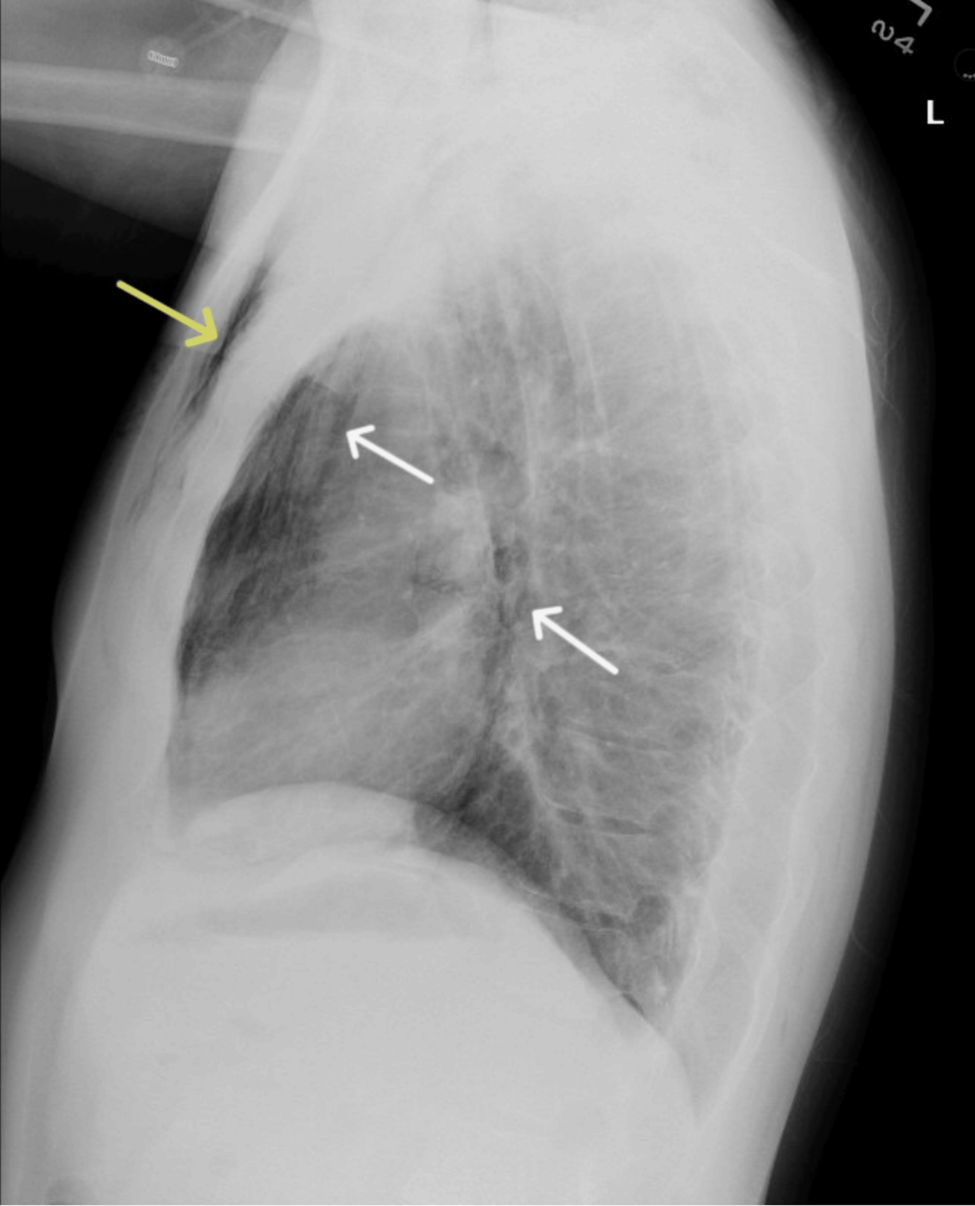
Lateral chest X-ray demonstrating pneumomediastinum (white arrows) and subcutaneous emphysema (yellow arrow).
